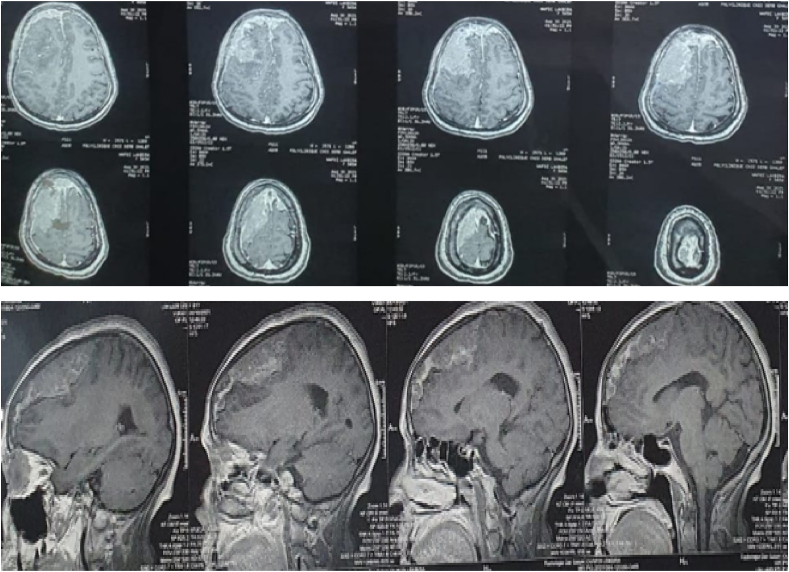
A brain MRI showed the same image with dural attachment on the right frontal lobe.
